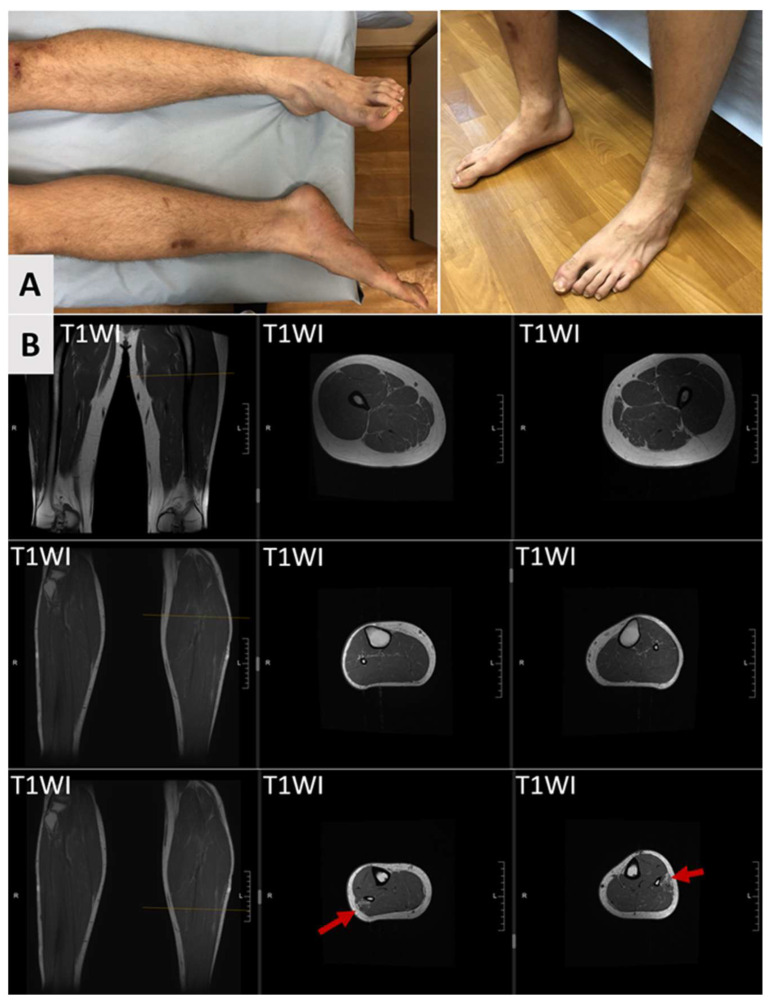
Clinical and lower limb muscle MR images of the proband. (A) Clinical presentation at age 26 years showing bilateral lower leg and foot muscle hypotrophy with pes planus. Also, arachnodactyly and sandal gap are noticed on both sides. (B) Coronal and axial T1-weighted MR images of lower limb muscles (age 20 years) demonstrate bilateral fat infiltration isolated to the peroneus longus muscles (arrows), with preservation of all other lower limb muscles.
